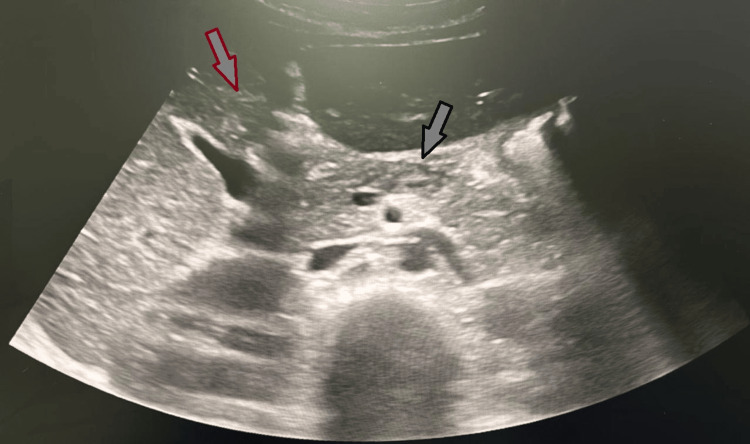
Transverse ultrasound of the abdomen. Ultrasound of the abdomen revealed mild splenomegaly (11 cm) and enlargement of the mesenteric lymph nodes in the right lower quadrant, while the liver and pancreas findings were unremarkable (black and red arrows, respectively).
